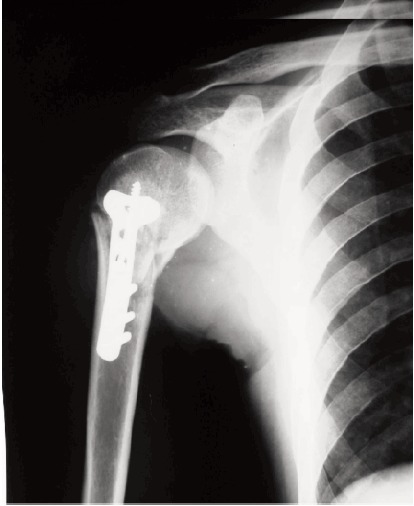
Fig. 3: Follow-up radiograph at 24 months. F3:
